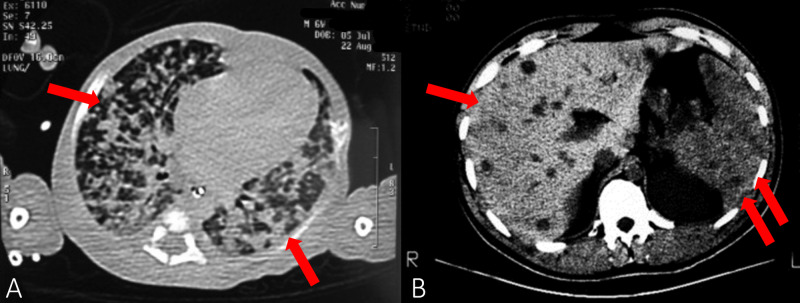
Axial CT. (A) Chest CT showing bilateral opacities. (B) Abdominal CT showing multiple nodular hepatic lesions (arrow) and enlarged spleen (double arrows).
